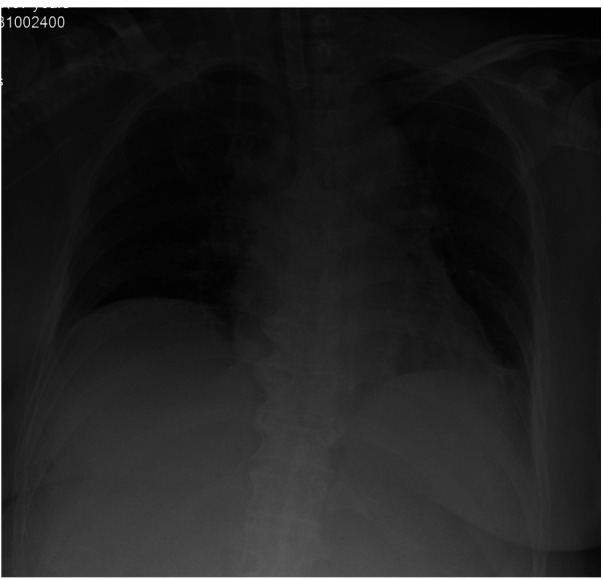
Chest x-ray revealed a normal position of diaphragm.
